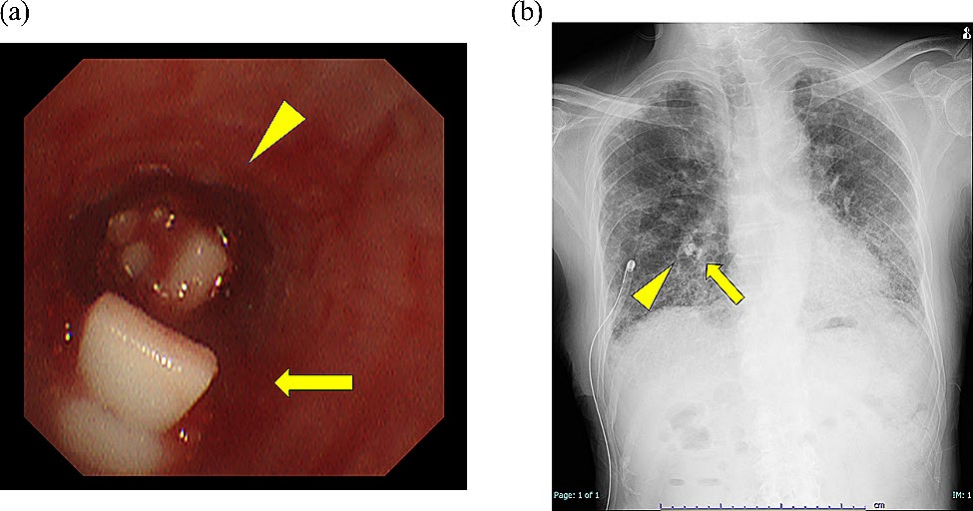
Endobronchial occlusion with silicone spigots. The combination of transbronchoscopic oxygen insufflation and a digital chest drainage system identified B5a and B5b as the bronchi responsible for the fistulas. B5a and B5b were occluded with size M (arrowhead) and S (arrow) spigots, respectively, and consequently the amount of air leakage decreased significantly (a: bronchoscopy image) (b: chest radiograph)
